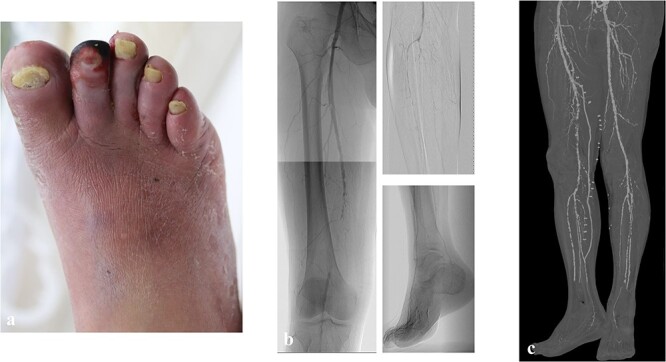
The preoperative clinical picture of case 1; (a) gangrene of the second digit with infection; (b) preoperative angiography; the GLASS stage was II (TAP: posterior tibial artery, FP2, IP2); (c) postoperative computed tomography; superficial femoral artery-posterior tibial artery bypass with a great saphenous vein was performed.
